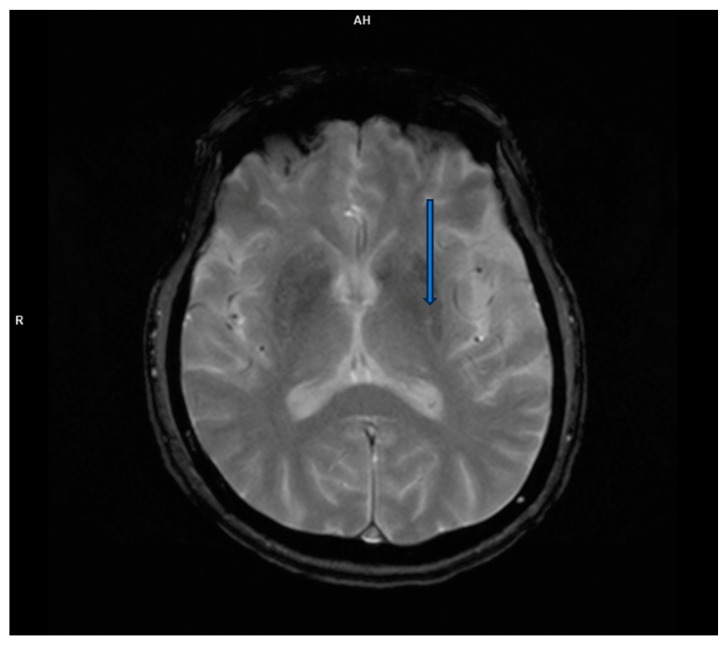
T2-weighted transverse MRI scan reveals enlarged perivascular spaces.
